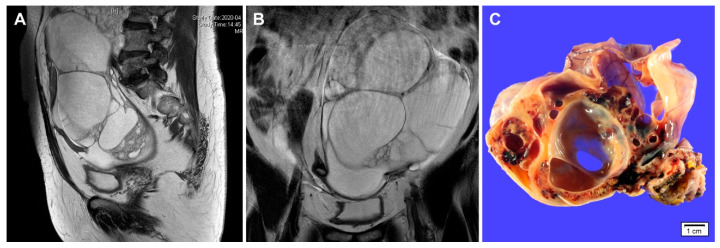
Imaging and gross findings of gynandroblastoma. (A,B) a 65-year-old woman presented with an abdominal mass. Abdominopelvic magnetic resonance imaging reveals a 20 cm multiseptated cystic mass arising from the left ovary. No evidence of abdominopelvic peritoneal seeding, lymph node enlargement, or hematogenous metastasis is identified. Preoperative laboratory test showed a slight elevation of serum CA 125 level (37.1 U/mL). The serum CA 19-9 level was within normal range (32.1 U/mL). (C) Grossly, the cut section of the ovarian tumor shows variable-sized, thin-walled cystic spaces. Solid areas show yellow-to-tan tumor tissue with hemorrhagic spots. The inner surface of cystic lesions is relatively smooth and glistening.
